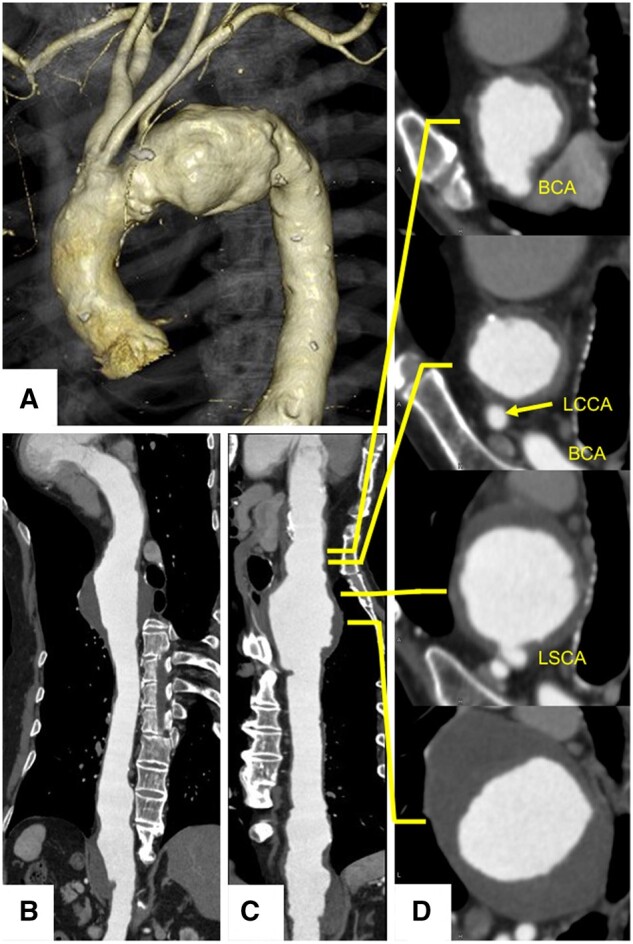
Three-dimensional computed tomography scan (A). A centreline image with curved multiplanar reconstruction was created (B). The curved multiplanar reconstruction image was converted to a straight multiplanar reconstruction (C). The thickness of the atheroma was evaluated from the origin of the brachiocephalic trunk to a section 2-cm distal to the left subclavian artery in the axial image (D) linked with the straight multiplanar reconstruction image. This patient is classified as grade 5 with the atheroma 2-cm distal to the left subclavian artery.
